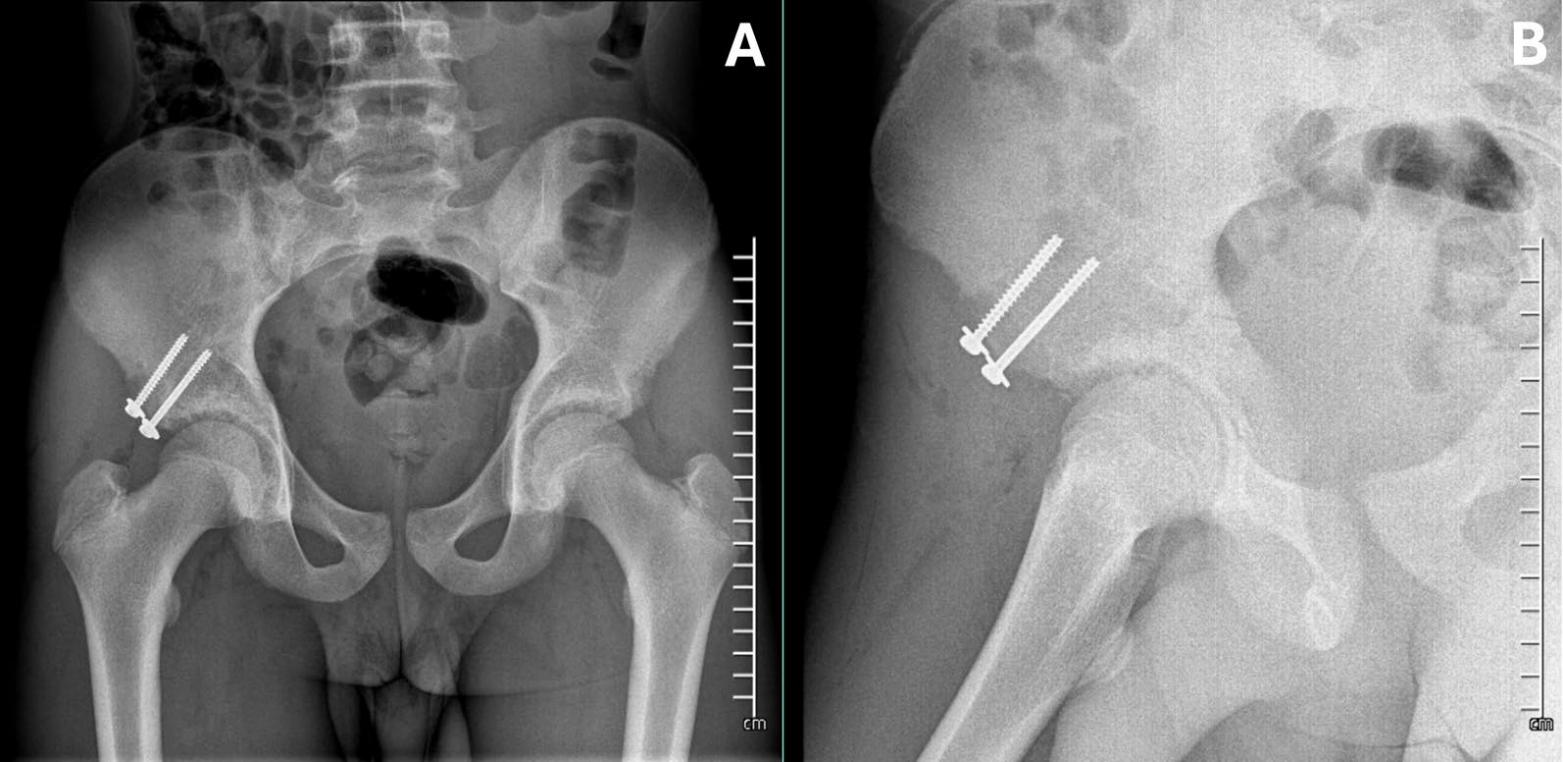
One-year follow-up x-rays in AP pelvis (A) and iliac wing (B) view showing proper screw placement, fracture healing, and no signs of heterotopic ossification
